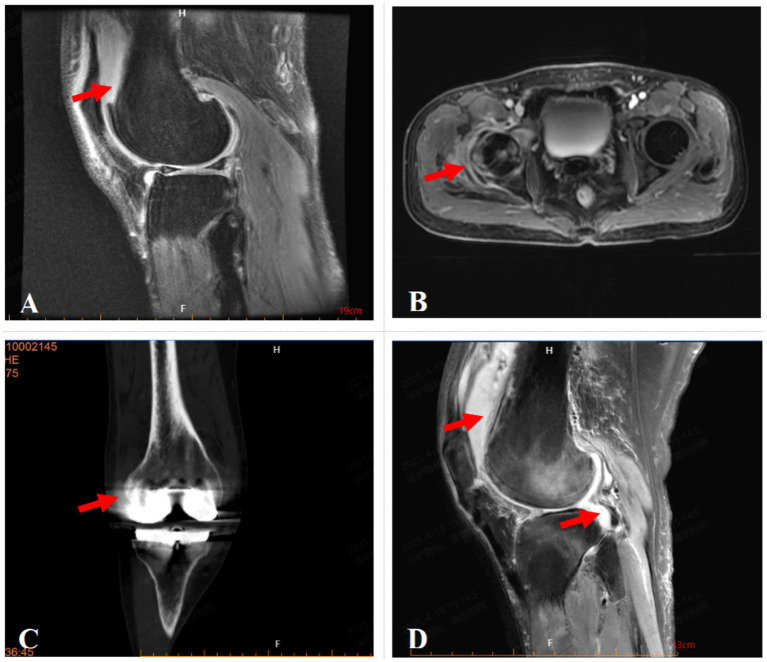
Imaging of the affected joints in four patients. (A) Fat-suppressed MRI image of case 1 shows anterolateral subcutaneous edema and suprapatellar bursal effusion. (B) Contrast-enhanced MRI image of case 2 demonstrates abnormal signal intensity with restricted diffusion and enhancement in the periaritcular soft tissues of the right hip, suggestive of inflammation with abscess formation. (C) CT image of case 3 shows Knee Prosthesis and swelling of the periarticular soft tissues. (D) Fat-suppressed MRI image of case 4 shows joint effusion and suprapatellar bursal fluid with periarticular soft tissue edema.
